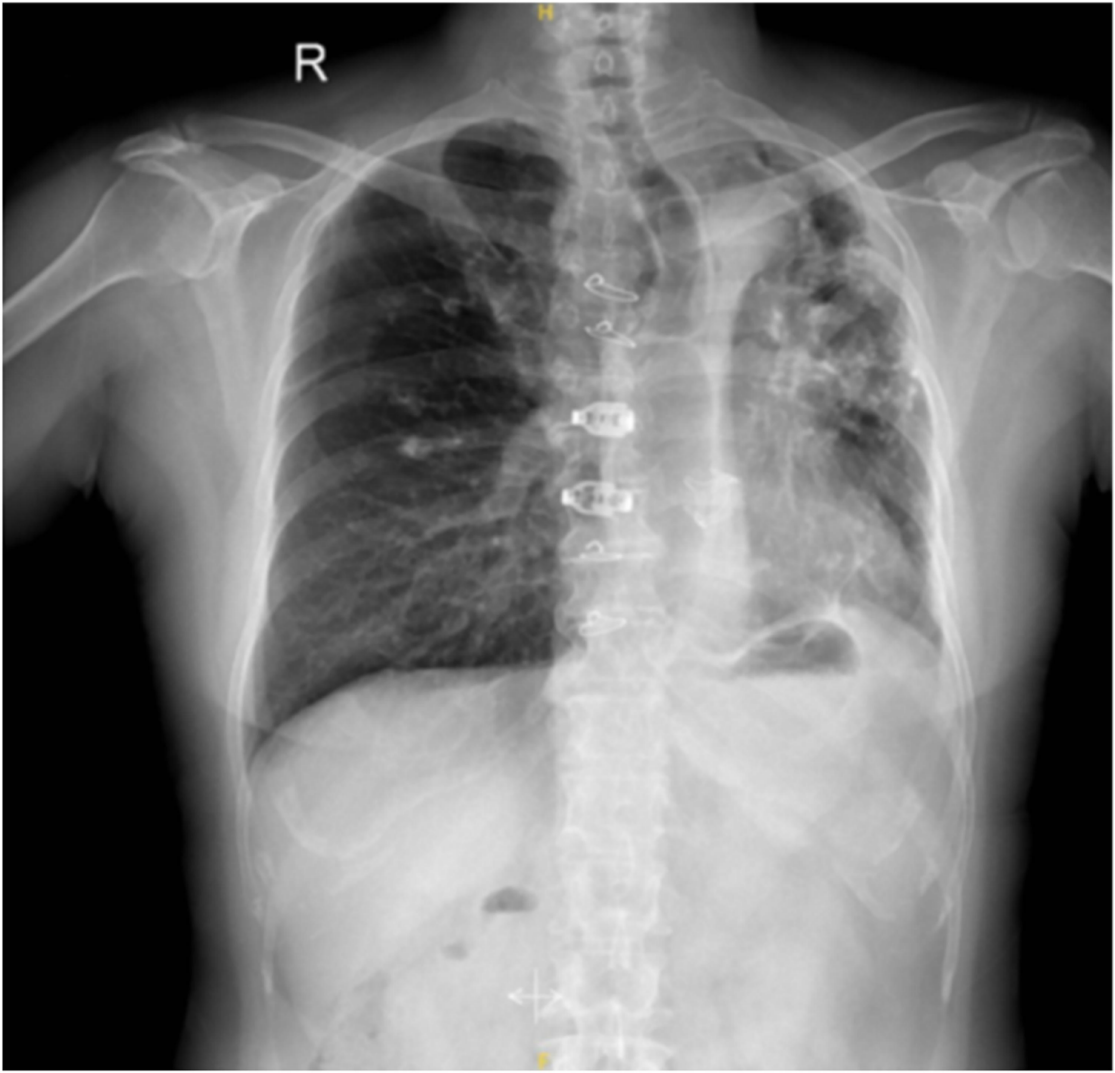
One year after operation, chest X-ray showed that the left lung reexpansion.
